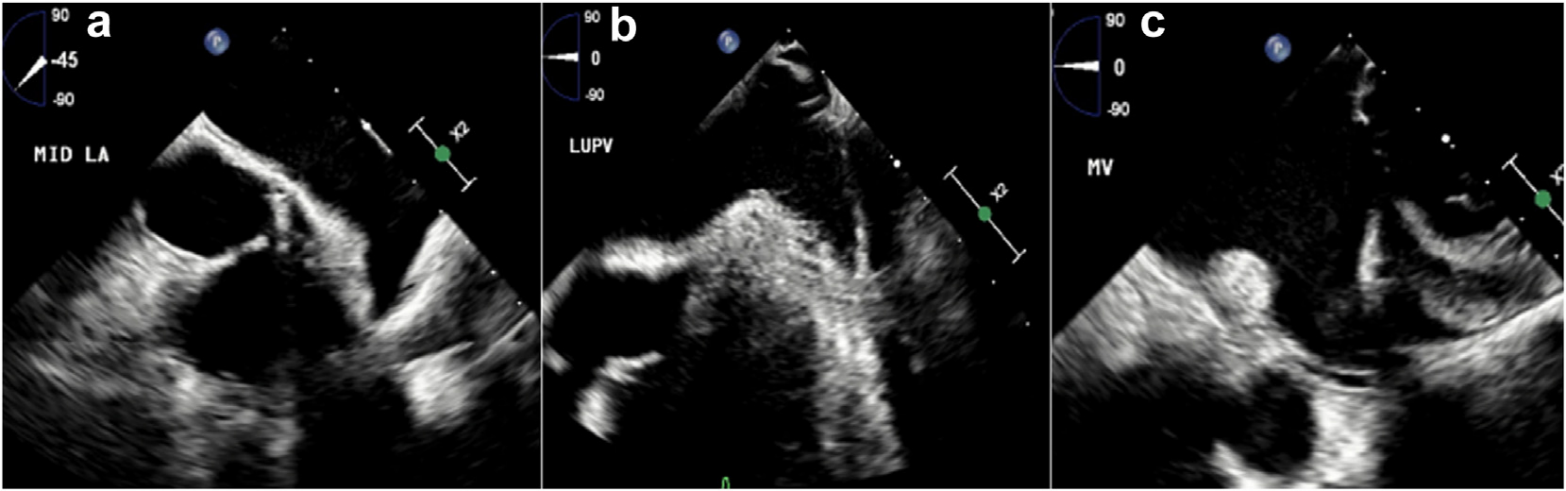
Key ICE imaging views of the LAA using 2D imaging. Panel A: mid-left atrial (LA) view. Panel B: left upper pulmonary vein (LUPV) view. Panel C: mitral valve (MV) or transmitral view Abbreviations: 2D, two-dimensional; ICE, intracardiac echocardiography; LAA, left atrial appendage.
